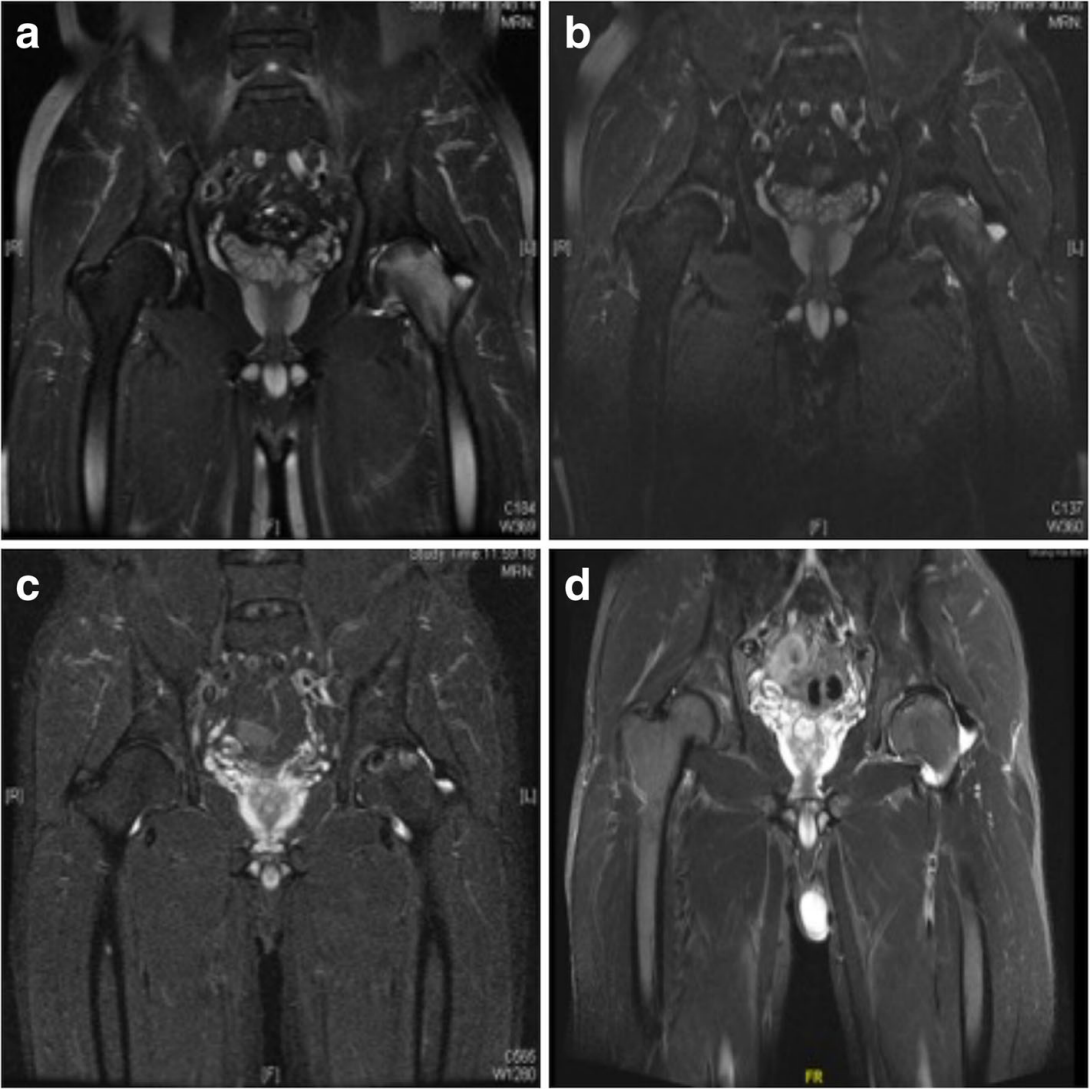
MRI of a mid-age man with long-term alcohol abuse: a MRI indicated ARCO stage II ONFH with grade 4 BME in the left hip before ESWT; b BME reduction was observed in 3 months after ESWT; c MRI in 5 years after ESWT; d MRI indicated grade 2 BME at the final follow-up. The patient has restored hip function without pain since 5 months after ESWT
